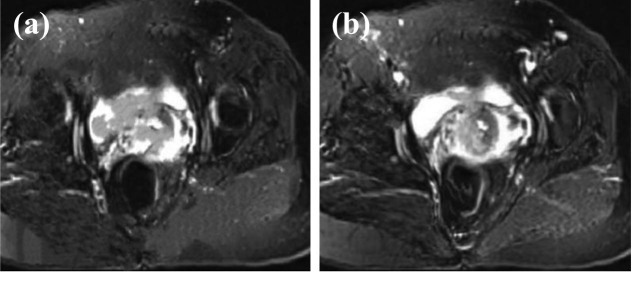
Comparison of MRI images before and after feature enhancement. (a) Original feature and (b) processed feature.
